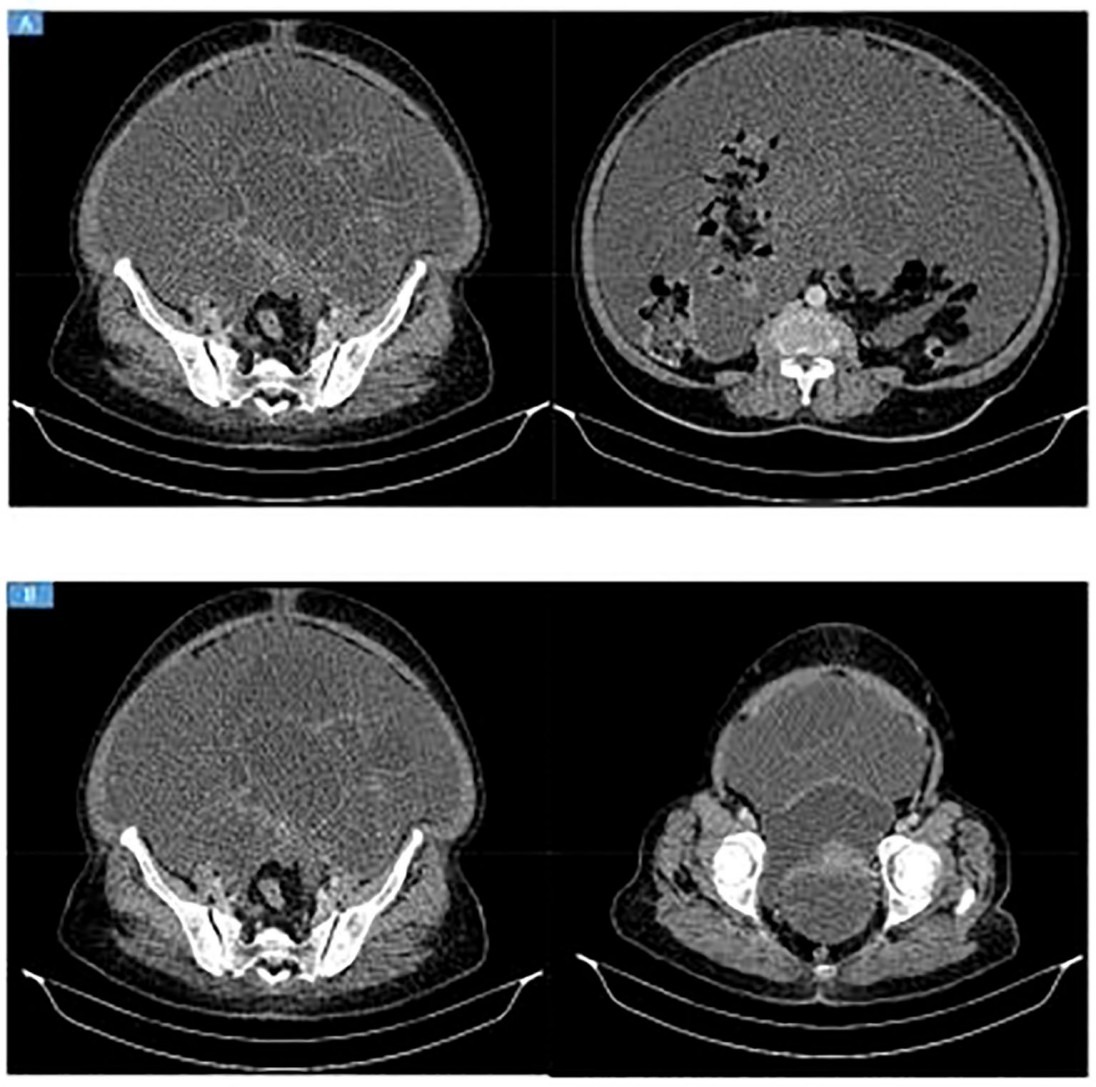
(A, B) Contrast-enhanced CT showing massive intra-abdominal fluid collectionwith thick, septated, enhancing areas predominantly in the lower abdomen and pelvis, associated with omental thickening and mild fat stranding. A large left adnexal lesionmeasuring approximately 20 × 12 cm is seen, containing enhancing solid components andinternal septations, causing posterior compression of the rectum. Findings are highly suggestive of ovarian malignancy, most likely mucinous cystadenocarcinoma.
